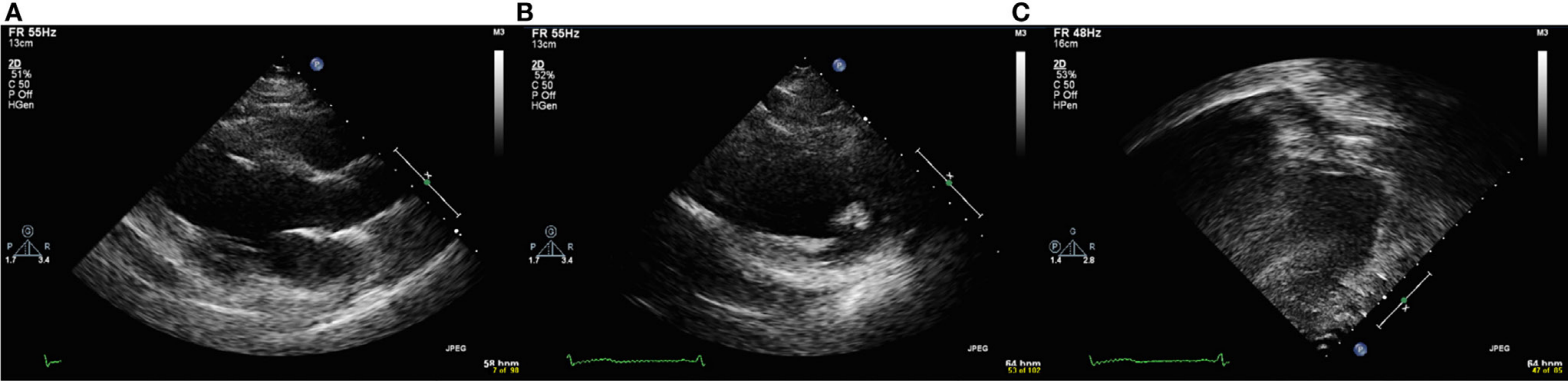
Echocardiographic images with poor endocardial border delineation from a single patient with Duchenne muscular dystrophy. (A) Parasternal long axis view. (B) Parasternal short axis view. (C) Apical four chamber view.
